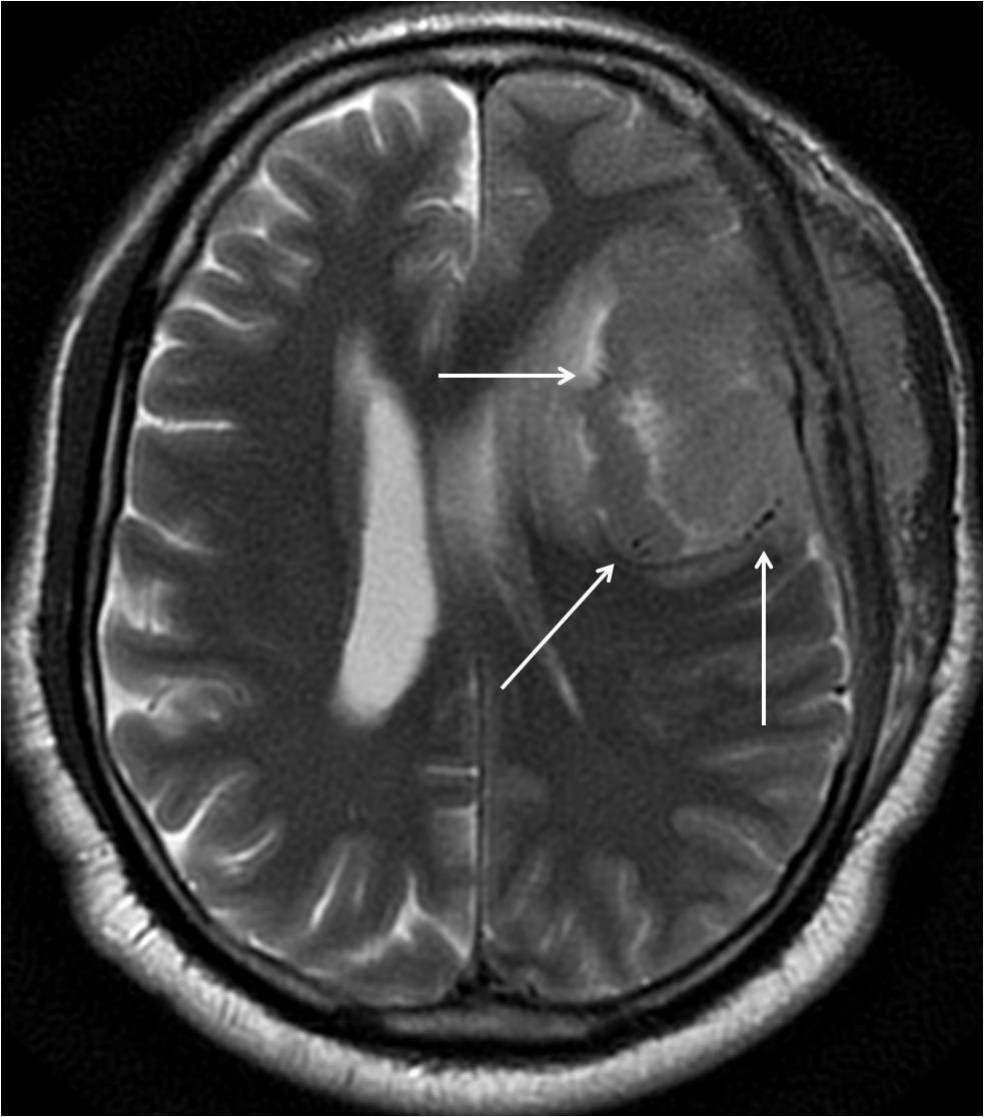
Axial T2 weighted magnetic resonance imaging (MRI) through the mass reveals multiple flow voids around the periphery of the tumor (arrows).
